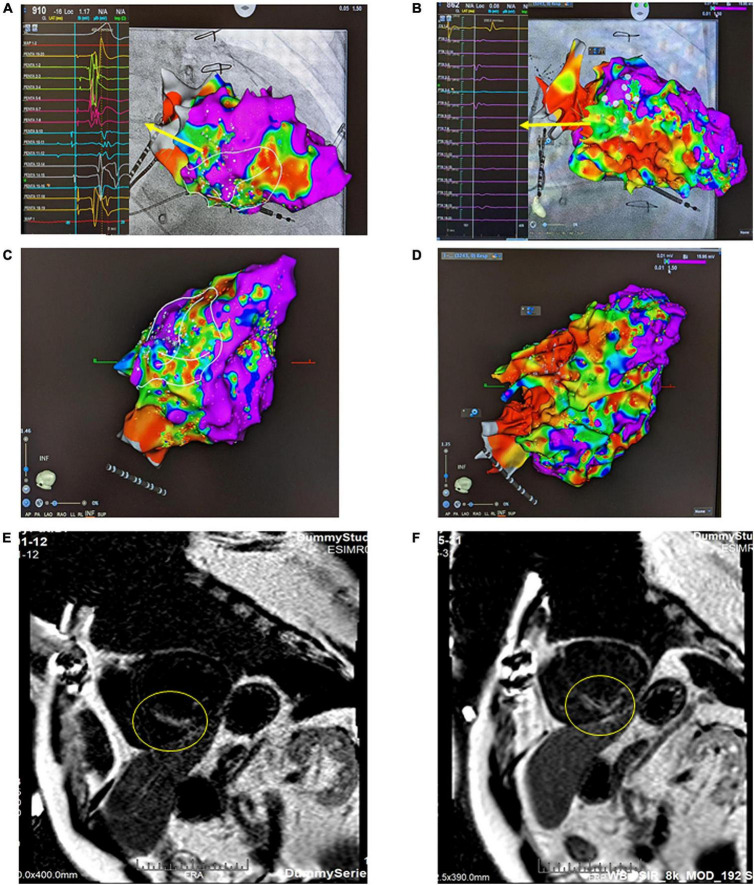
Bipolar voltage mapping with a 3D CARTO mapping system (Biosense, Diamond Bar, CA). (A) The presence of a large, uneven, mixed scar and viable zone in the area of the left ventricular basal to the middle posteroseptal wall from the right anterior oblique (RAO) view. The arrow indicates LAVA, the substrate, as the target ablation zone. (B) After SBRT, the therapy zone became a scar zone. The arrow indicates local voltage < 0.5 mV. (C) Inferior view of the left ventricle (LV) before SARS. (D) Inferior view of the left ventricle (LV) after SBRT. (E) Cardiac MRI using a wide-band sequence before SBRT. A delay in the enhancement of the mid-basal septal wall and mid-inferior wall was noted, which indicated scar formation in this area. (F) Cardiac MRI after SBRT. The previously shown delay in enhancement of the mid-basal septal wall and mid inferior wall was still present; however, the affected area was slightly larger than that on the previous MRI.
